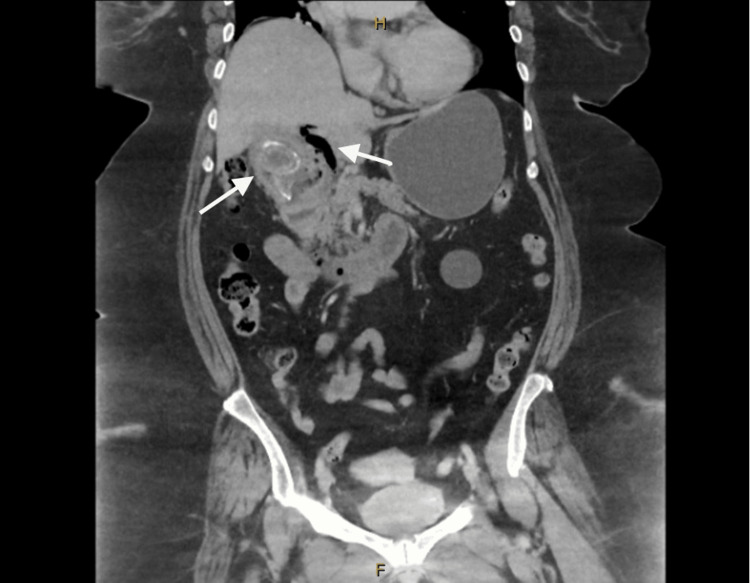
CTAP with IV contrast slices demonstrating 4 cm gallstones and pneumobilia in the left intrahepatic bile duct (Case 1) CTAP: computed tomography abdomen pelvis, IV: intravenous
